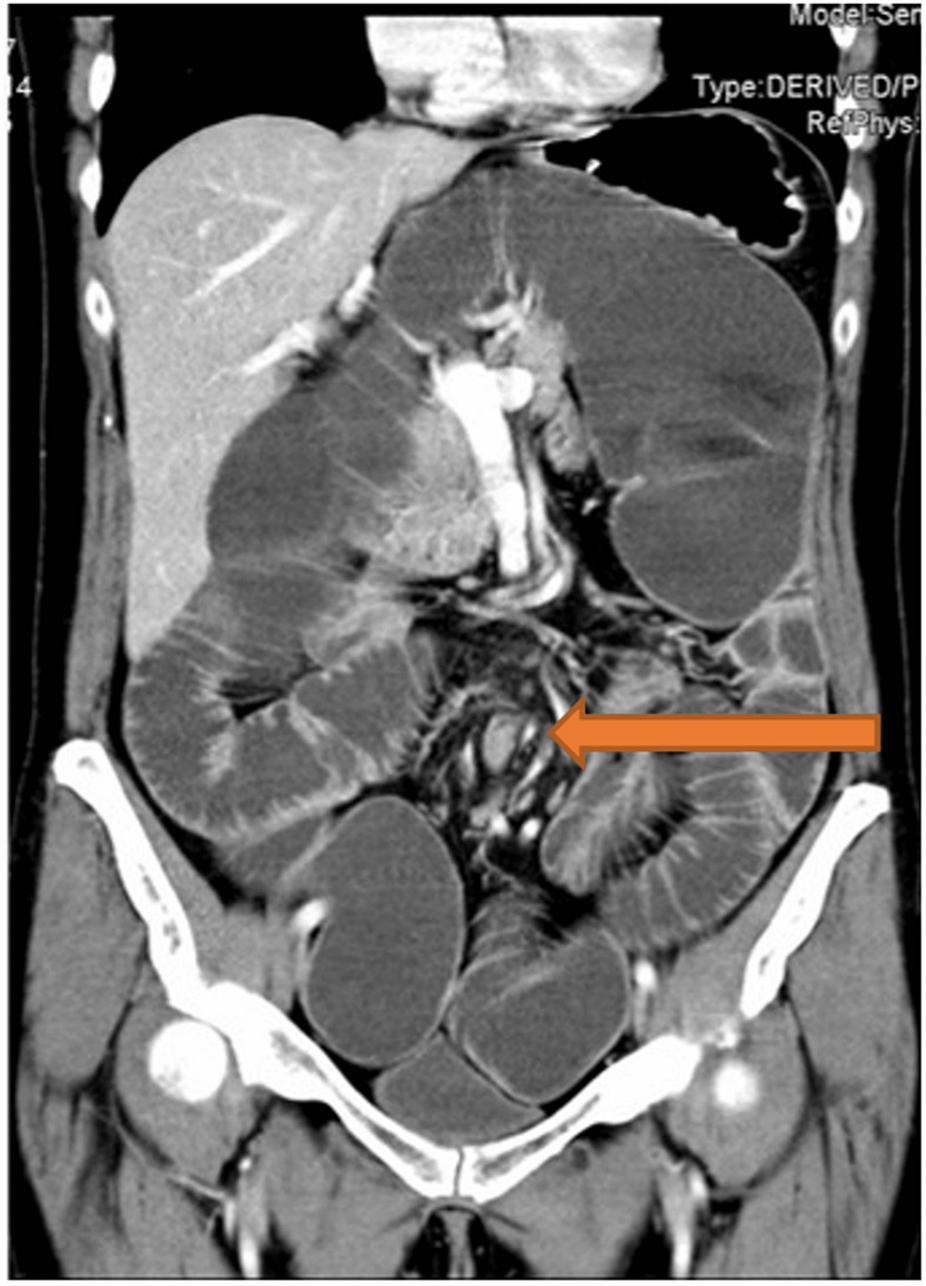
CT scan demonstrating the "whirlpool" sign of the mesentery (arrow) seen in pouch volvulus CT: computed tomography
